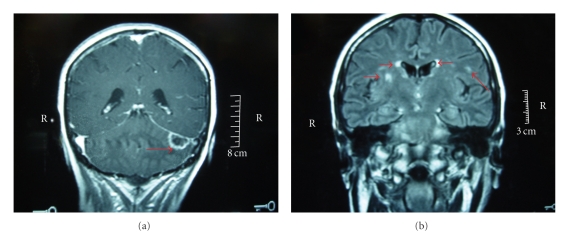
Cerebral MRI: (a) coronal view T1 with gadolinium injection: left cerebellar nodular lesion with central necrotic zone and peripheral contrast enhancement. (b) Coronal view T1 without gadolinium injection: periventricular localization of multiple cerebral hypersignal nodular lesions.
